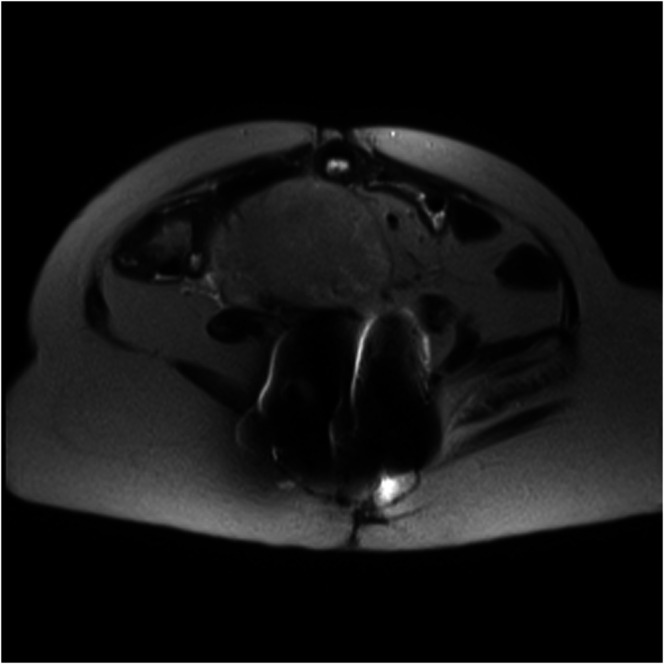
An enlarging pelvic mass on abdominal and pelvic magnetic resonance imaging.
